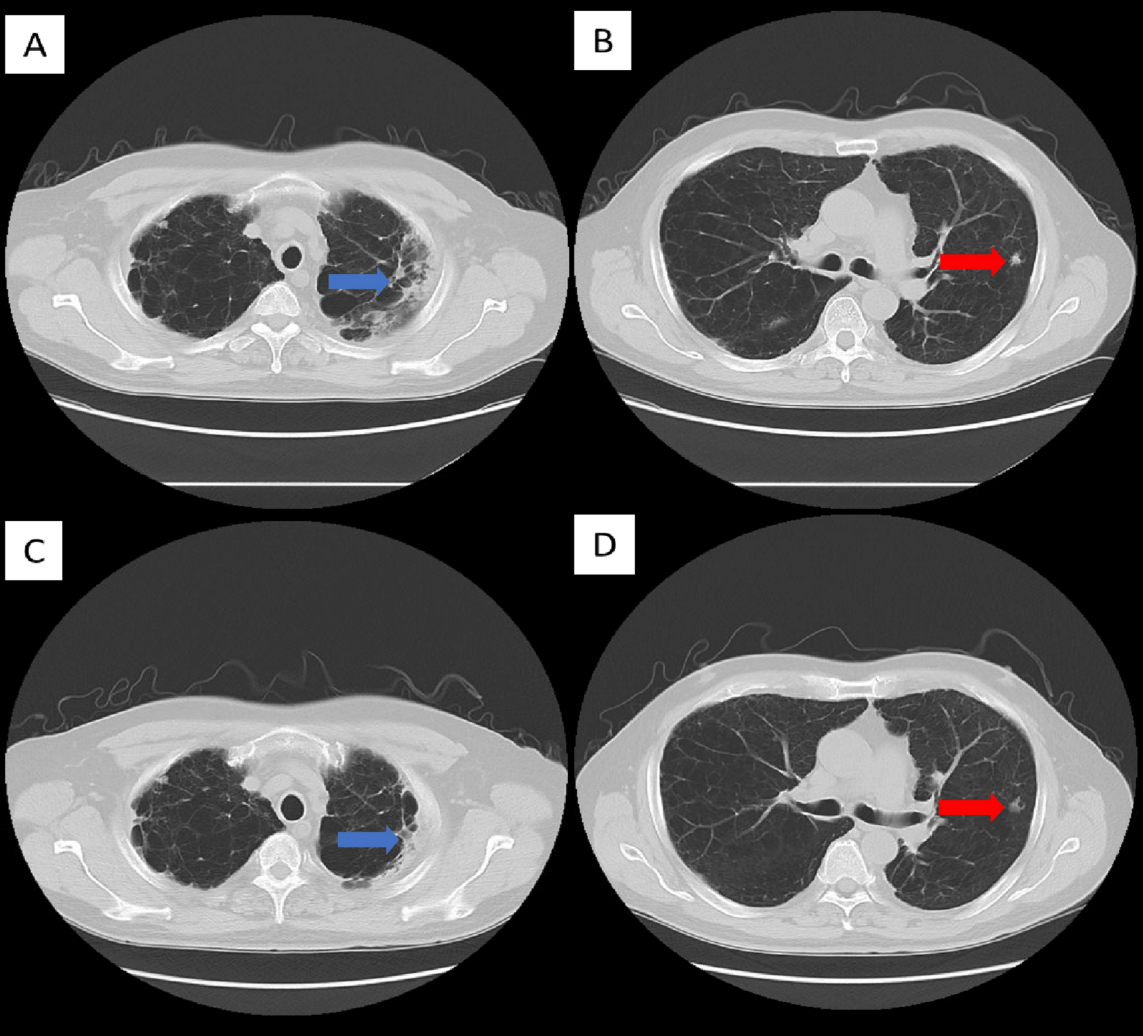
The CT scans of the patient at the time of admission (A, B) and after recovery (C, D) The images demonstrate that infiltrates from meningococcal pneumonia improved with treatment (blue arrows) and there were persistent findings of amyloid nodules (red arrows). Background emphysematous changes were also evident.
